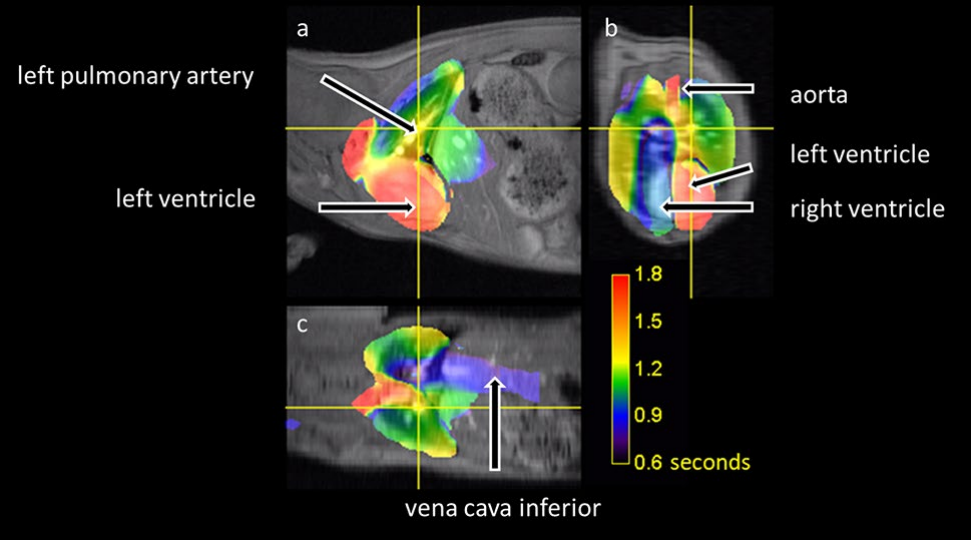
Bolus arrival time map fused with MRI in (a) sagittal, (b) transversal and (c) coronal perspective. The presence of the tracer bolus in the right ventricle starting at about 0.9 s is shown in blue colors and in the left ventricle appears approximately 0.8 s later in red color. Lung perfusion is shown in green and yellow colors representing the time interval in between.
